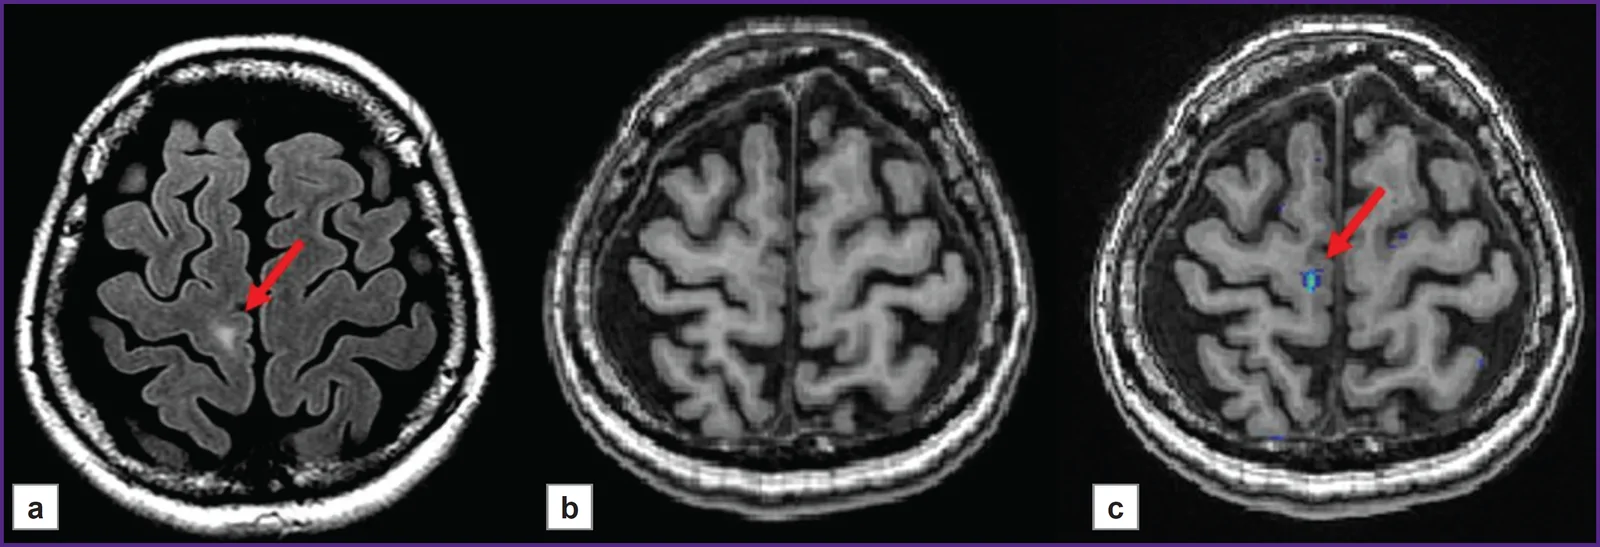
MRI of a 45-year-old woman with focal cortical dysplasia in the right precentral gyrus: (a) structural MRI (T2/FLAIR) with the arrow pointing to the lesion area; (b) classical morphometry: no abnormalities detected; (c) multimodal morphometry: clear dysplasia zone highlighted, with the arrow indicating the area of change
